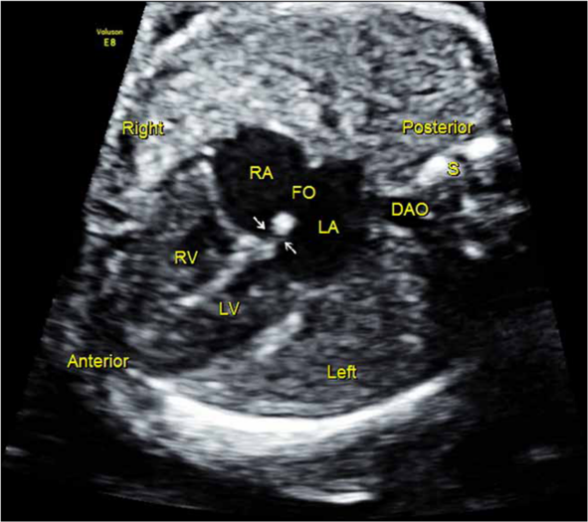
Sonographic images of a 21-gestational-week fetus in which PAVSD was falsely suspected. In the 4CV, a deficiency of the atrial septem primum was suspected by sonographers under Protocol A, as indicated by the white arrows. It was in actuality the echo of the coronary sinus which could be visualized when a non-standard 4CV was obtained with the section across the atrioventricular groove. These specific signs were mentioned in Protocol B
